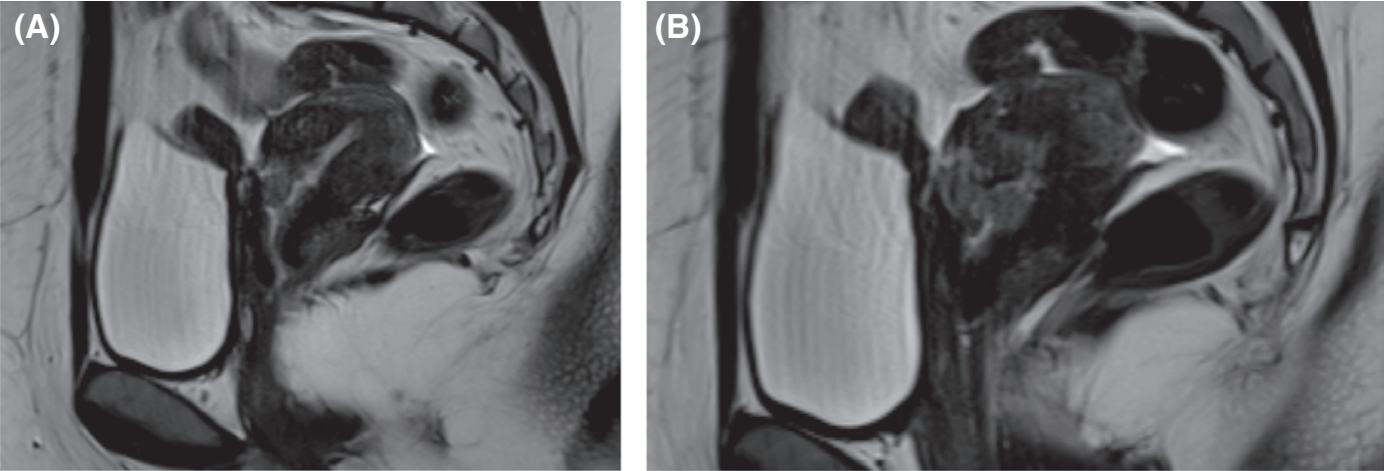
A and B, The appearance of a tumoral mass (recurrence) in the sagittal section of pelvic MRI on the CS scar line in the three months after chemotherapy
